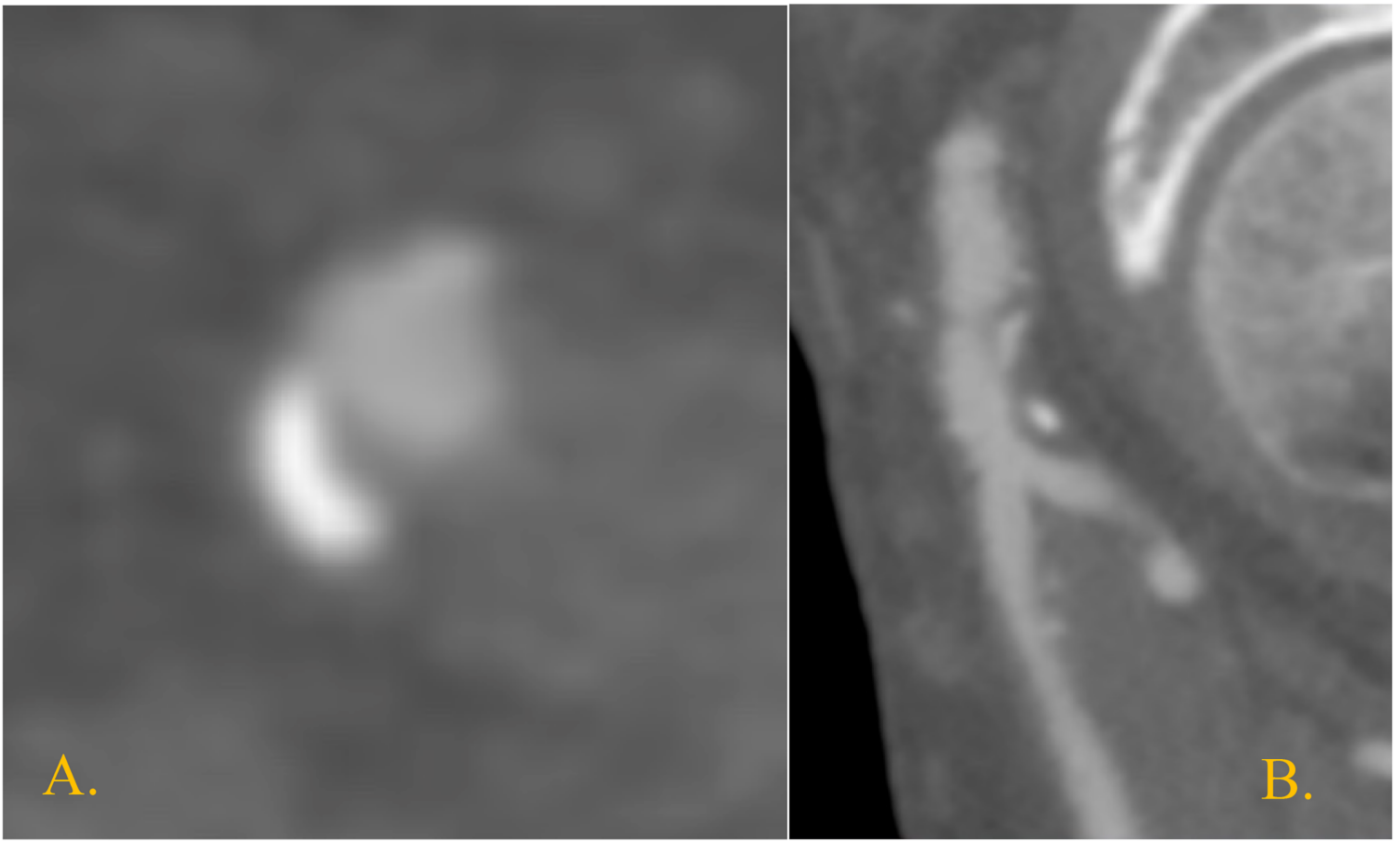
Right CFA at preoperative CTA in the only case of postoperative focal dissection. It shows a posterior atheroma plaque at the level of the endovascular access (A) transversal section; (B) sagittal section.
